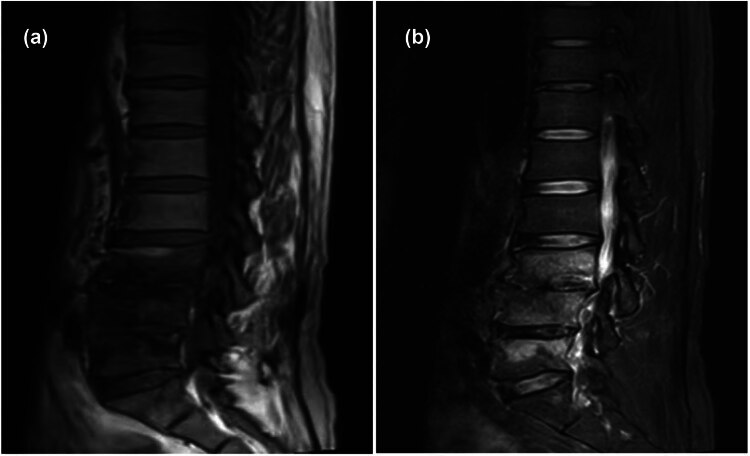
Lumbar spine MRI indicates abnormal signal intensity in the L3–L5 vertebral bodies with low signal on T1-weighted images (a) and high signal on T2-weighted images (b), suggestive of an infectious lesion.
